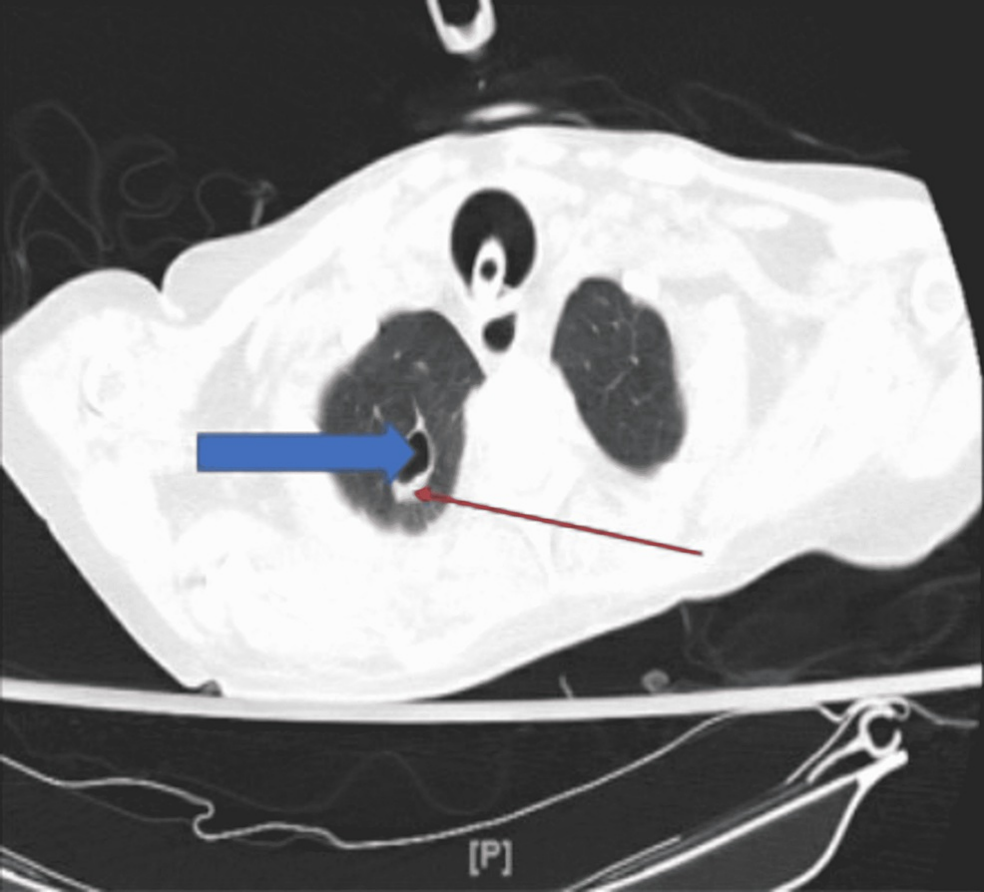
CT showing a thin-walled cavitary lesion in the apical segment of the right upper lobe. The red arrow is pointing to the thin walls of the cavitation, and the blue arrow is pointing to the cavitation as a whole.
